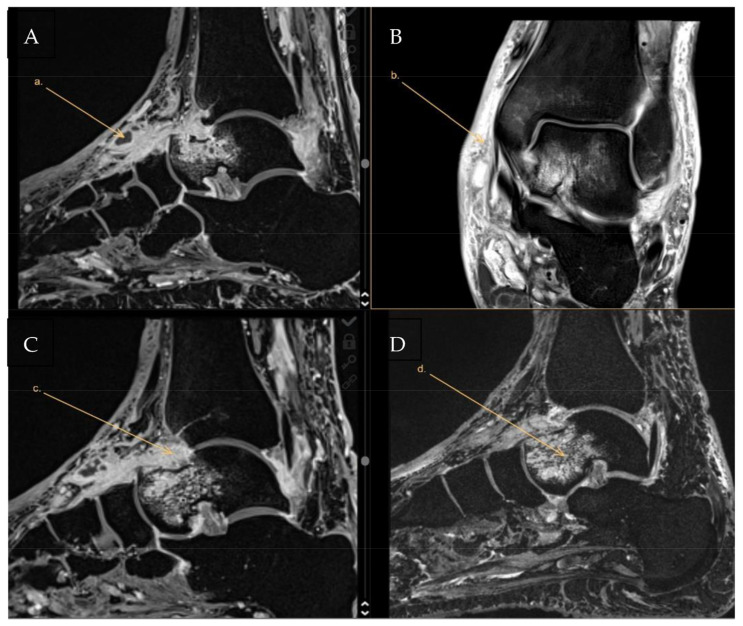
MRI of the affected left ankle on day 8. Arrows in the individual panels show (A) multiple small abscesses in the medial side of the feet, (B) edema of the tendons, (C) arthritis of the TC joint, and (D) osteomyelitis of the talus.
